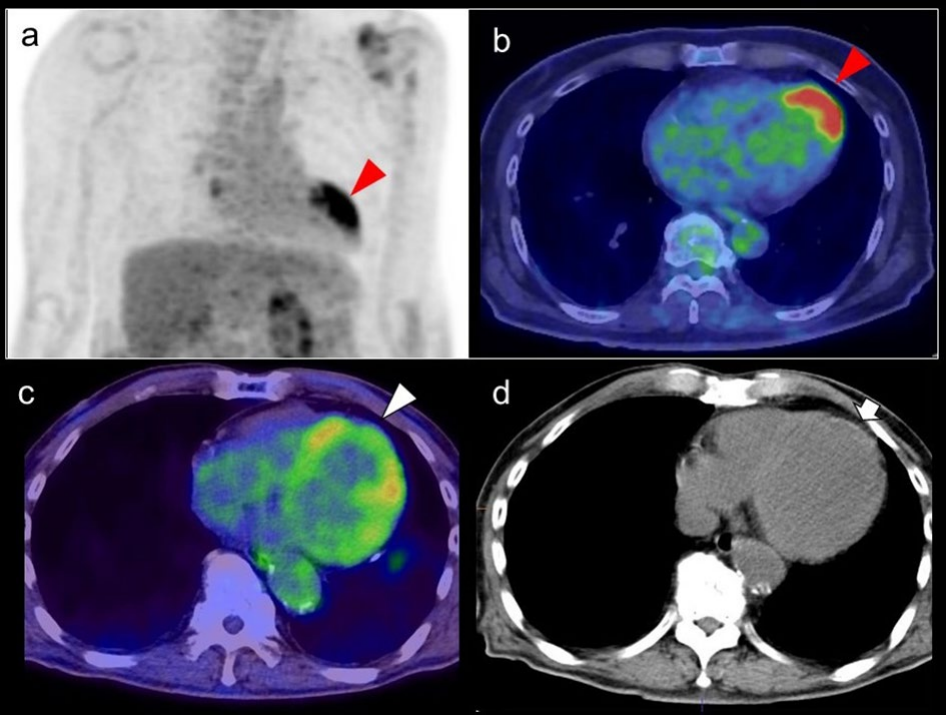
Myocardial ischemic change. Focal FDG uptake in the anterior of myocardium (a, b red arrowheads) had a possibility of ischemic change, which is caused by metabolic switch from fatty acid to glucose use. Whereas defect of FDG uptake at apex (c white arrowhead) with slight calcification on CT (d white arrow) suggest chronic myocardial infarction
